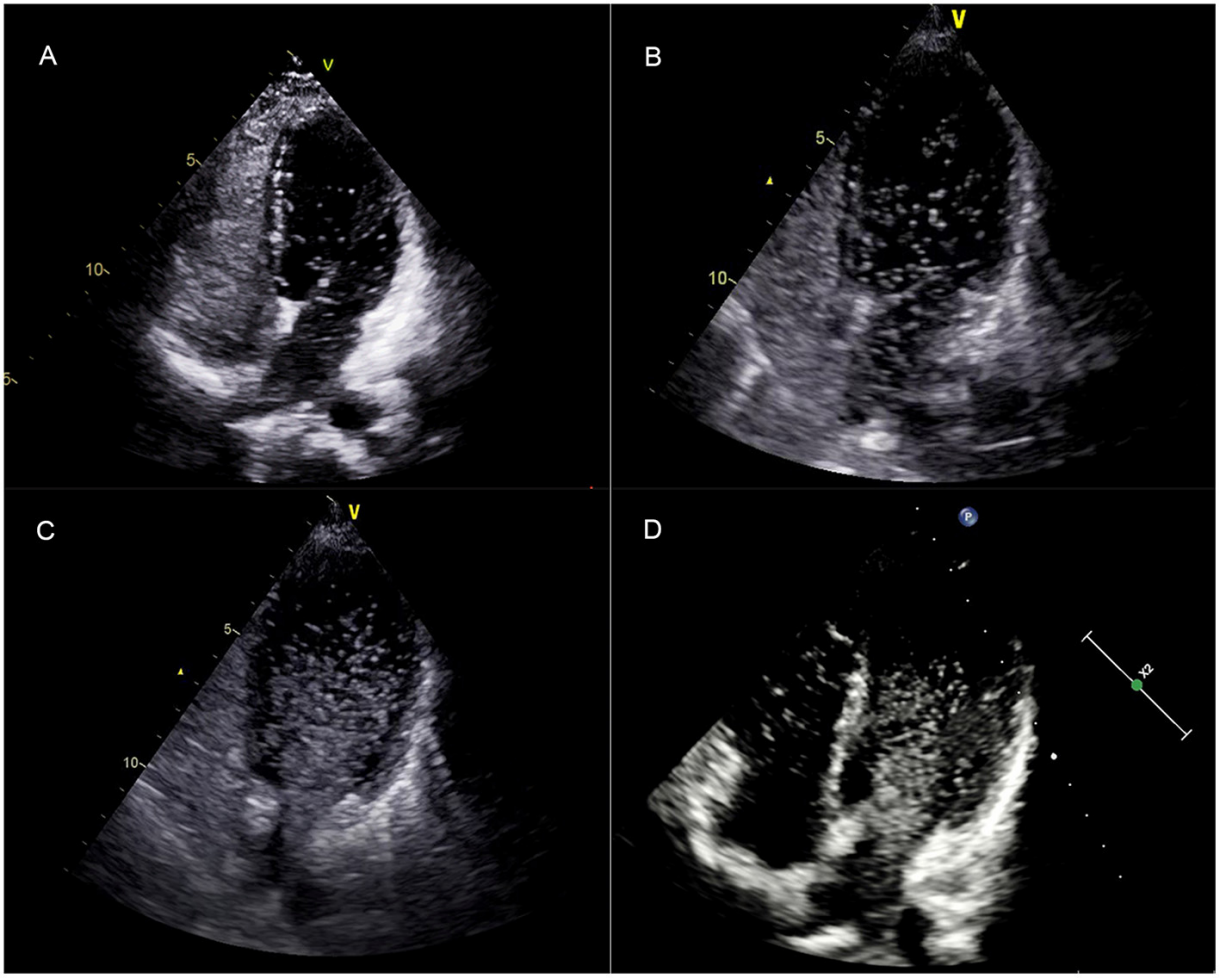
After complete opacification of the right atrium, the TTTE showed the microbubbles in the left chamber after five cardiac cycles in a single frame image. (A–C) correspond to a mild (grade 1), moderate (grade 2), and extensive (grade 3) pulmonary RLS, respectively. (D) showed that the left chamber microbubbles lasted until the right chamber microbubbles disappeared. TTCE, transthoracic contrast echocardiography; RLS, right to left shunt.
